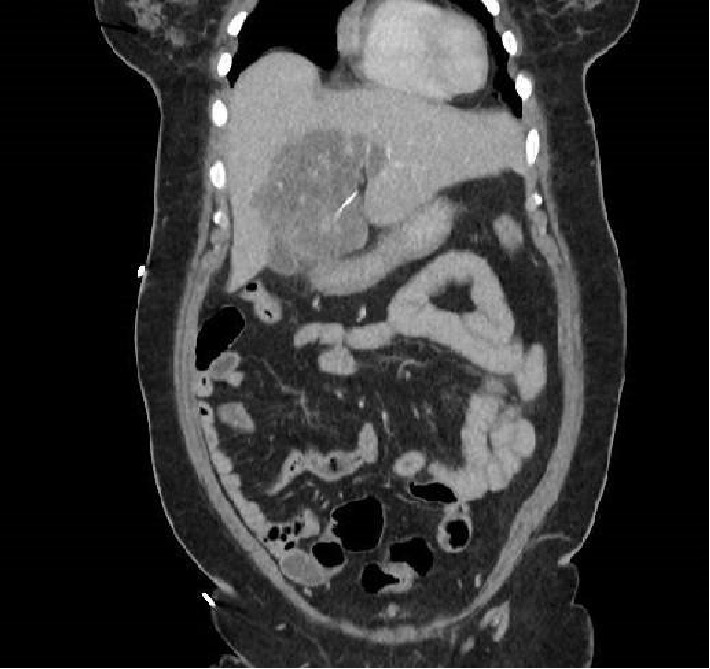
Computed tomography of the abdomen and pelvis with foreign body.
